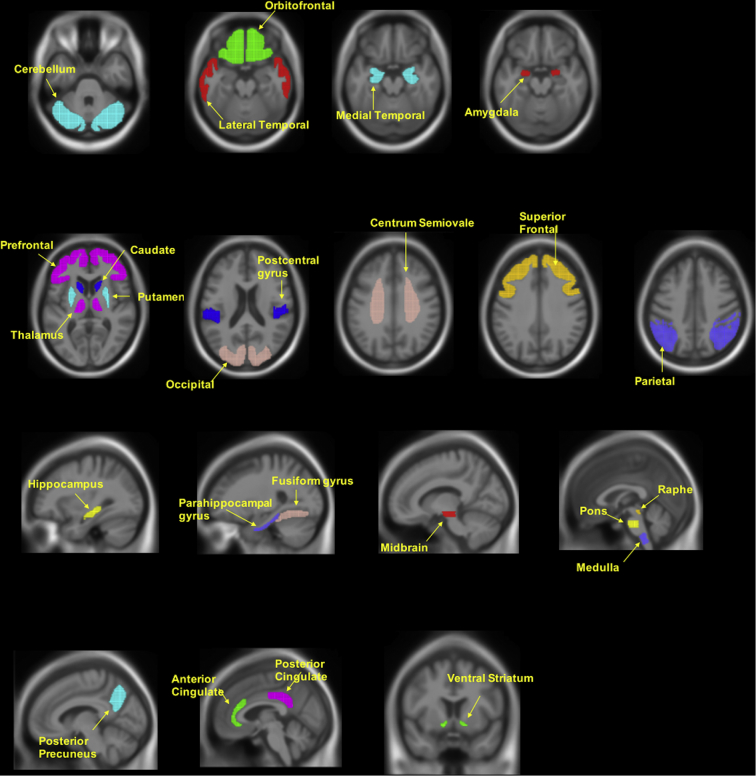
ROIs drawn superimposed on standard MNI152 MRI template. Representative ROIs are shown drawn on the MNI152 template using PMOD. ROI SUVRs were obtained by applying the above ROIs to SUVR images in the MNI space.
